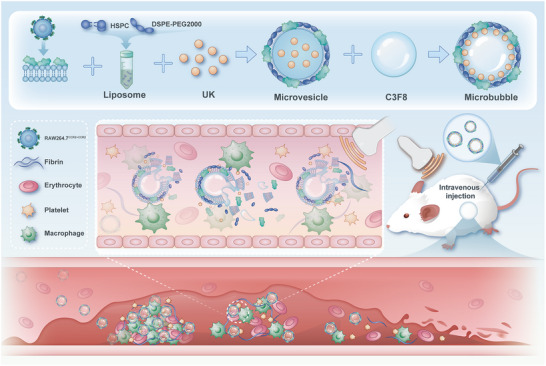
Design and mechanism of CCR2‐functionalized biomimetic microbubbles. Engineered macrophage membranes overexpressing CCR2 are used to construct urokinase‐loaded microbubbles (UK@CCR2/MBs). The microbubbles actively target the thrombus via the MCP‐1/CCR2 axis, where the localized ultrasound triggers payload release for precise thrombolysis.
